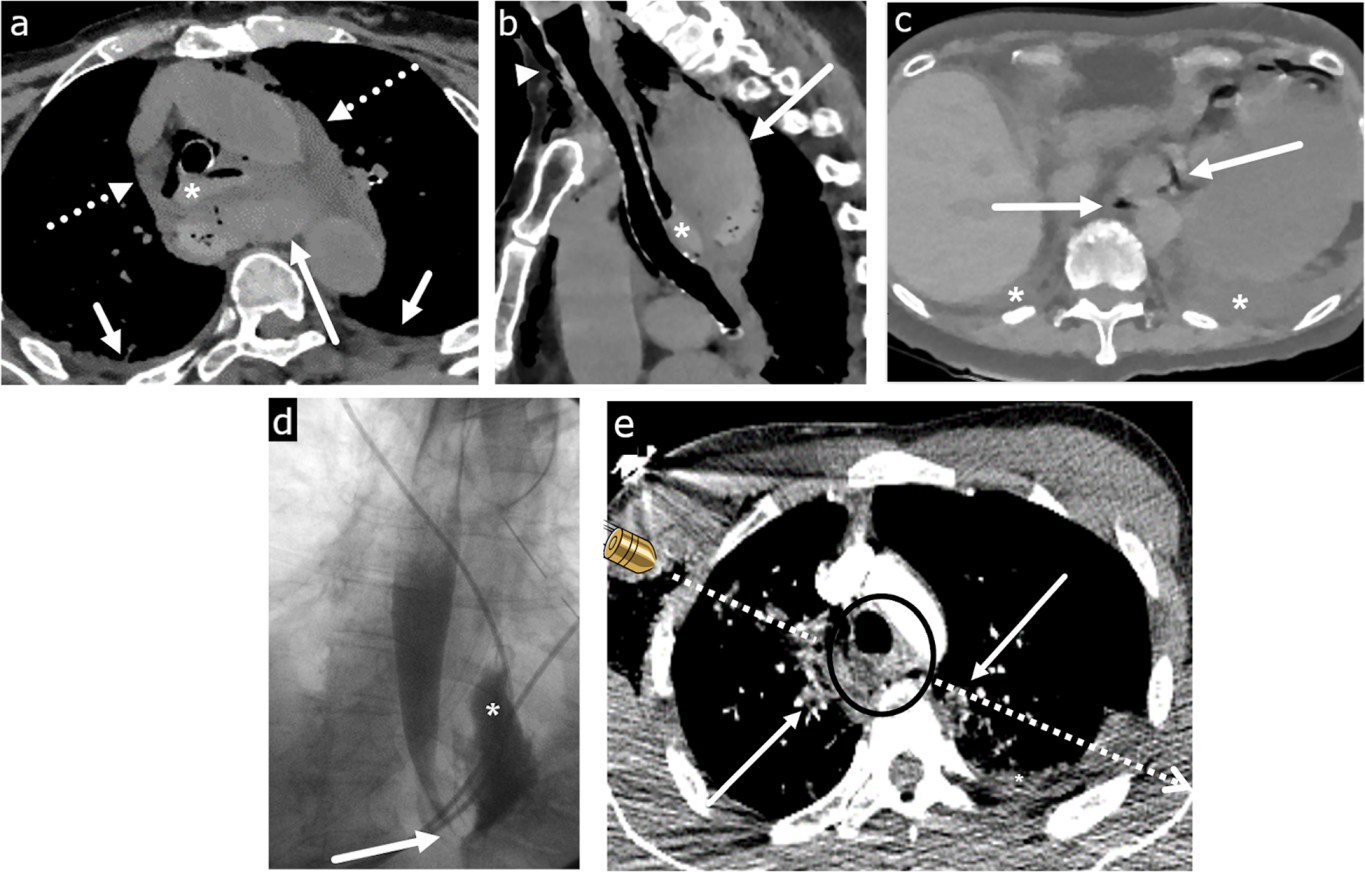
Esophageal perforation. a, b Iatrogenic esophageal perforation. Eighty-year-old woman with chest pain after transesophageal echocardiography. Axial (a) and sagittal (b) CT images show a dilated and blood-filled upper esophagus (asterisk) contiguous with a large posterior hematoma containing multiple locules of gas (long arrow). Extensive hemo-pneumomediastinum (dashed arrow), small bilateral pleural effusions (short arrow), and subcutaneous emphysema in the lower neck (arrowhead) are seen. c, d Boerhaave syndrome. Eighty-seven-year-old woman with epigastric and back pain after forceful vomiting. c Axial CT image at the gastroesophageal junction shows extraluminal gas surrounding the lower esophagus (arrow). Bilateral pleural effusions (asterisk). d Single contrast esophagography demonstrates luminal narrowing and irregularity of lower esophagus (arrow) with large contrast extravasation into the left pleural space (asterisk). e Traumatic esophageal perforation. Twenty-five-year-old male with gunshot wound to the chest. Axial CT image shows a bullet tract from right axilla to the left upper hemithorax (dashed arrow) resulting in bilateral pneumothoraces (not shown), left hemothorax (asterisk), and pulmonary contusion and laceration in the bilateral upper lobes (arrow). There is esophageal thickening and indistinctiveness with a small hematoma (circle) representing esophageal injury/perforation
